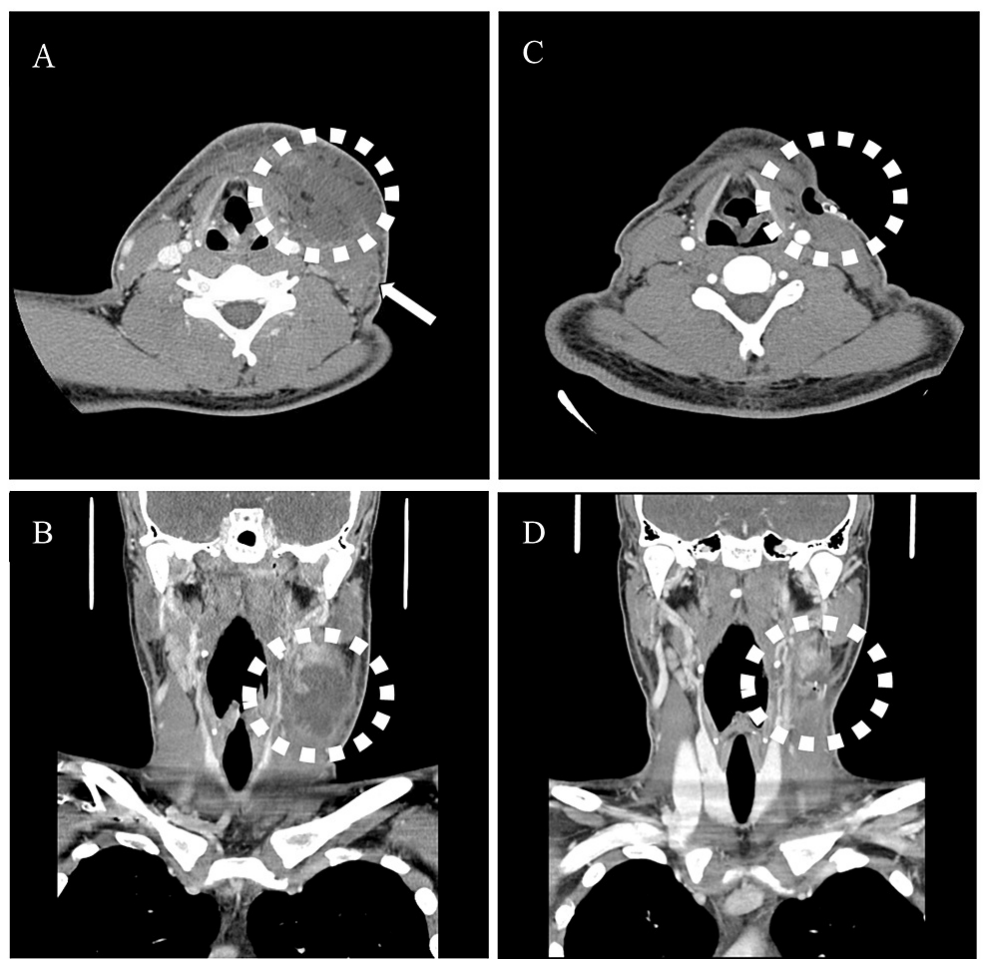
Preoperative (day one) and postoperative (day three) CT images of deep neck abscess and thrombophlebitis in the left external jugular vein. (A) Preoperative CT axial view, (B) Preoperative CT coronal view, (C) Postoperative CT axial view, (D) Postoperative CT coronal view ⇨: Thrombophlebitis of the left external jugular vein
